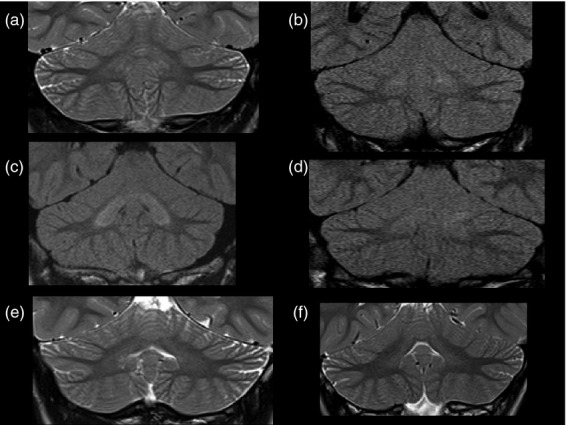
Patients with EAST syndrome show increased signal intensity of the cerebellar nuclei and cerebellar hypoplasia on brain magnetic resonance imaging. Coronal T2 or fluid-attenuated inversion recovery images show varying degrees of signal hyperintensity in the cerebellar deep nuclei. These ranged from subtle signal changes in comparison with the cerebellar cortex to more obvious changes within both the cerebellar deep nuclei and hilar white matter. (a) patient 1-1, (b) patient 2, (c) patient 4, (d) patient 3, (e) patient 5-1, and (f) patient 5-2.
